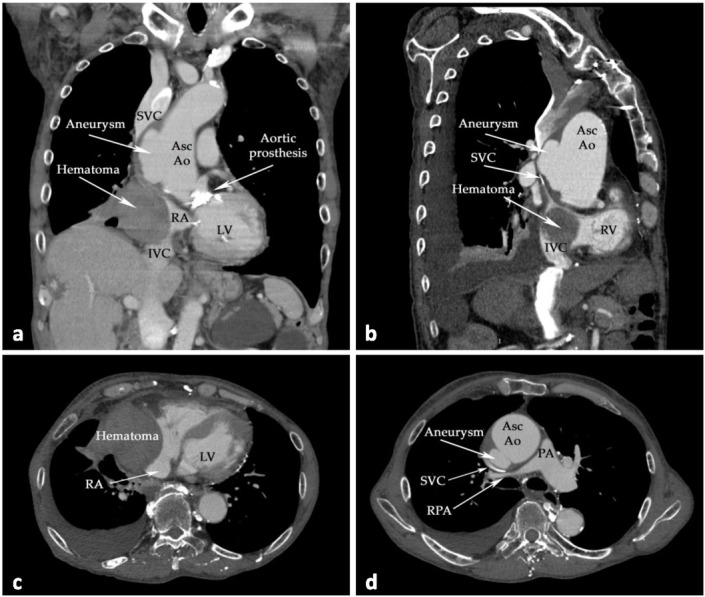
Emblematic thoraco-abdominal CT scans. In the coronal and sagittal scans ((a) and (b), respectively), the presence of an ascending aortic aneurysm, hematoma, and compression on RA, SVC, and IVC can be observed. (c) Compression of RA; (d) compression of SVC and RPA (axial CCTA scans). Abbreviations: AscAo—ascending aorta; IVC—inferior vena cava; LV—left ventricle; PA—main pulmonary artery; RA—right atrium; RPA—right pulmonary artery; SVC—superior vena cava.
